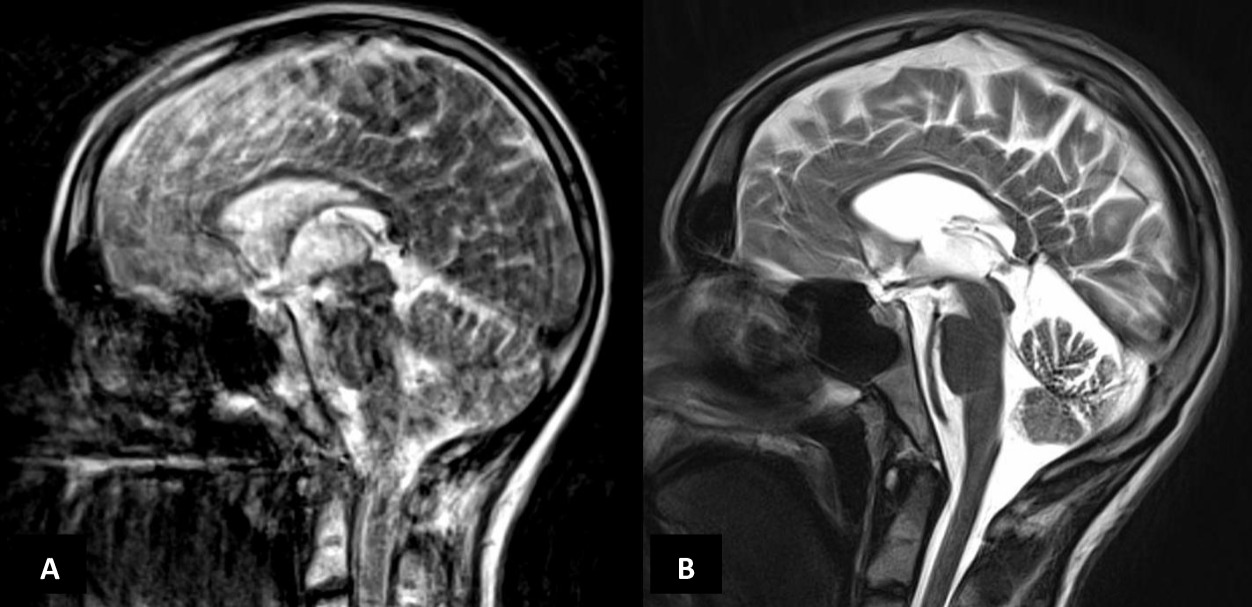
(A) MR scan of brain (T2-weighted sagittal) in 2012 (image quality degraded by movement artefact) and (B) a corresponding section from 2018 showing prominent cerebellar and corpus callosal atrophy.
